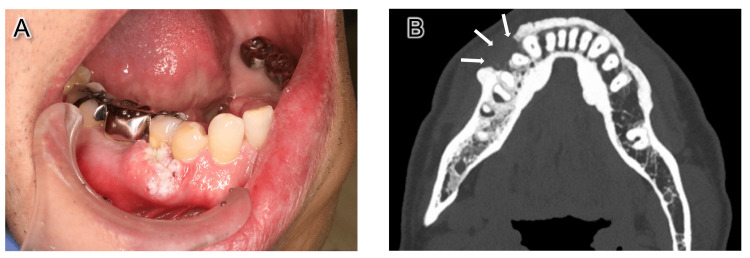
Intraoral photograph and CT image. (A) Clinical examination showed a mass measuring 20 × 15 mm in the right lower premolar region. (B) CT image of the tumor area. Bone invasion was seen (white arrows).
